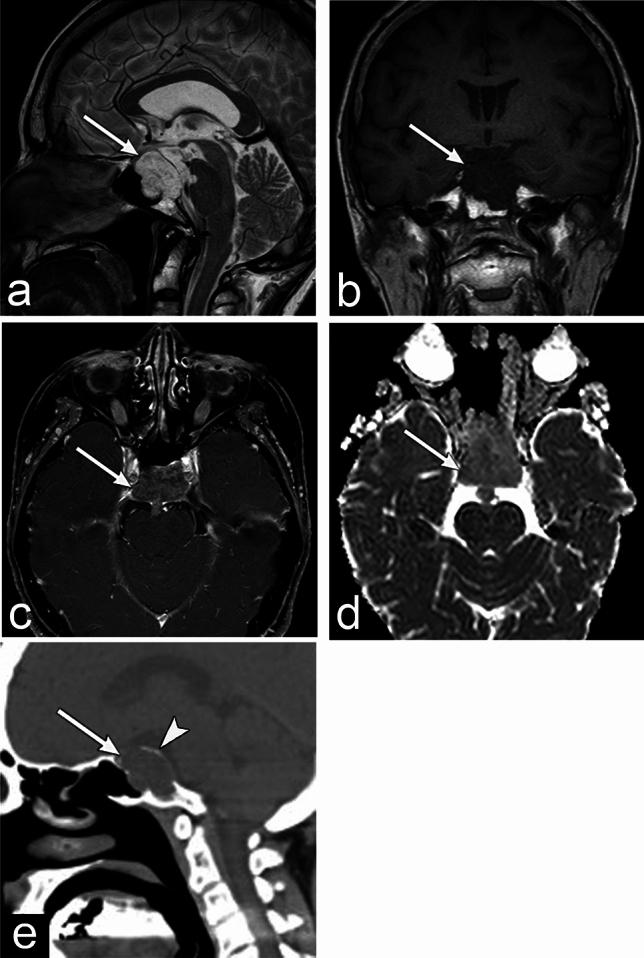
Chordoma of a woman in her 50s with bilateral diplopia. a–d MRI shows a well-defined lobulated mass (arrow) protruding upward from the midline of the clivus. The mass shows slightly heterogeneous high signal intensity on the T2 weighted image (WI) and homogeneous low signal intensity on T1WI with heterogeneous mild enhancement. The apparent diffusion coefficient value is 1.11 × 10^–3^ mm^2^/s. e CT shows a low attenuation mass (arrow) with destruction of the clivus. Lenear high attenuation structure (arrowhead), which appears to be residual bone, is observed at the margins of the mass
